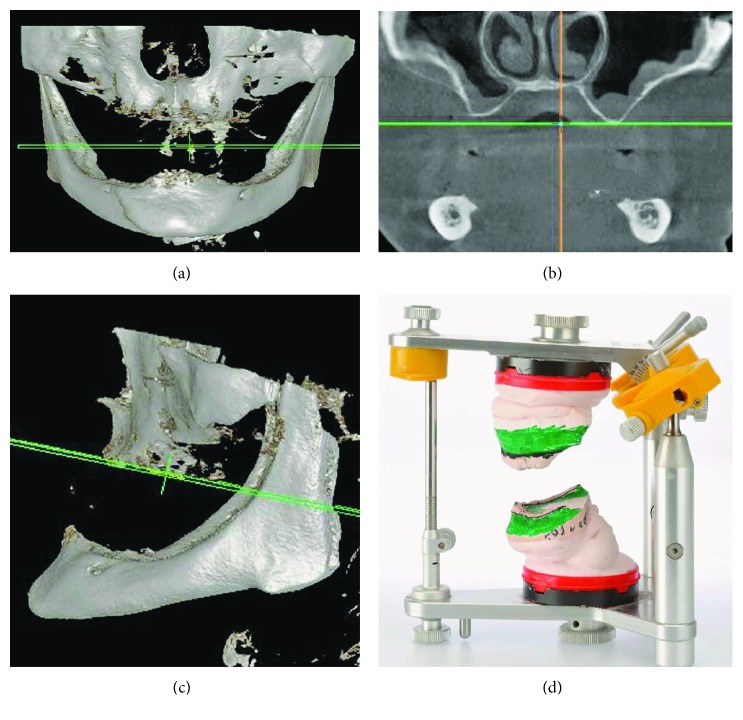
CBCT bone masking showing generalized advanced atrophy of the maxilla and posterior mandibular atrophy: (a) front view; (b) sinuses in sagittal section; (c) side view; (d) articulated casts show patient's jaw relationship.
